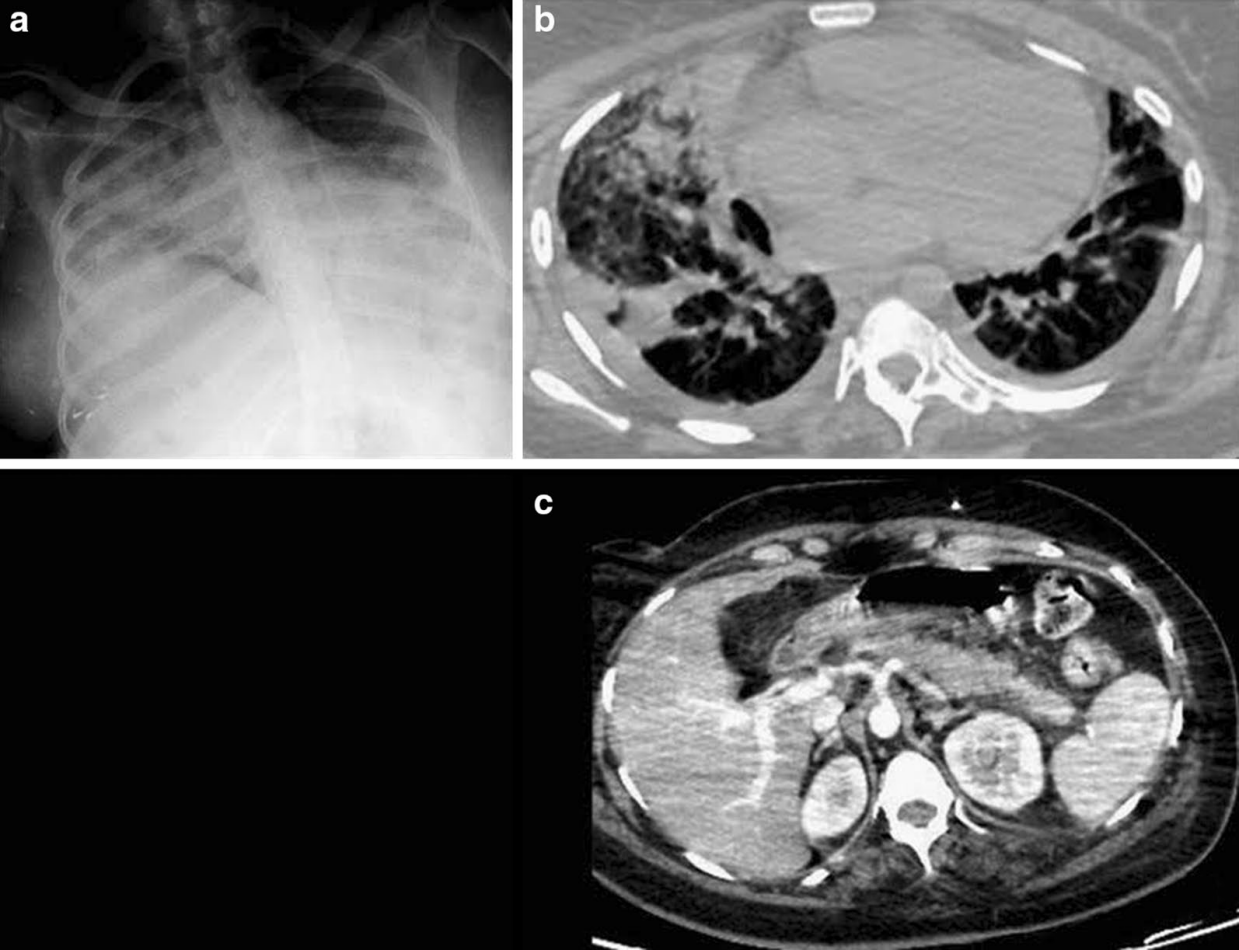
a Chest radiograph showing diffuse alveolo-interstitial infiltrates. b Chest computed tomography scan showing diffuse parenchymal consolidations combined with ground glass opacities. c Abdominal computed tomography scan showing acute interstitial edematous pancreatitis without necrosis, grade C on the Balthazar score
